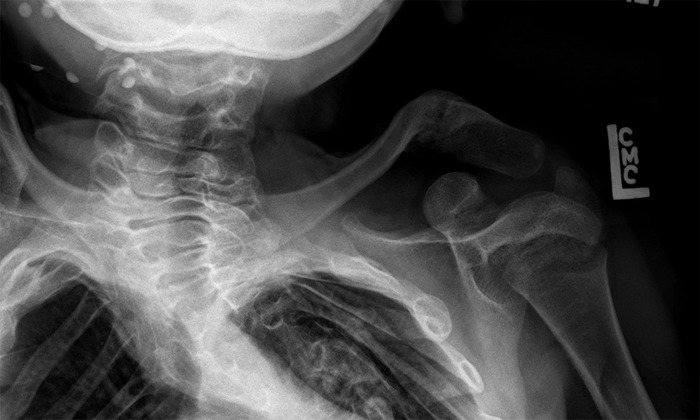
Radiograph of the left shoulder girdle of the female sibling at age 5 showing abnormally shaped and hypoplastic clavicle, scapula, and proximal humerus.
